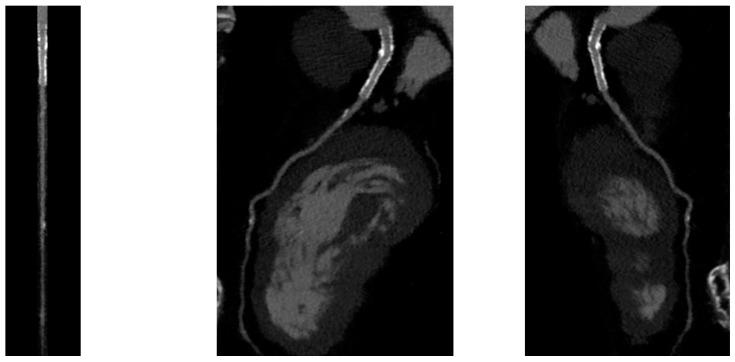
CCTA assessment of left main drug-eluting stent (Lumen image on the left, multiplanar reconstruction in the center and on the right). With a new CT scan, beam-hardening artifacts are reduced, and the stent lumen can be assessed with good performance.
